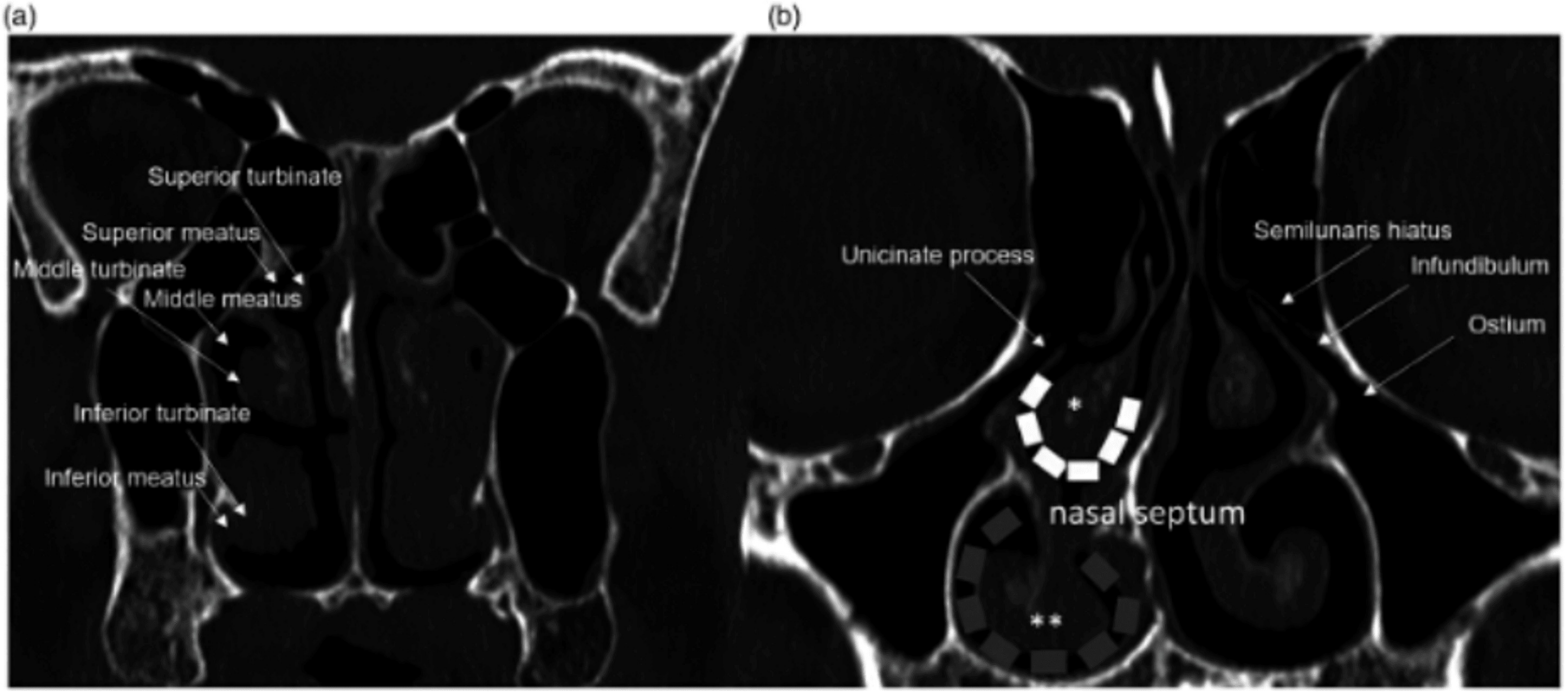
Coronal CT views of the nasal cavity anatomy and turbinate-related obstruction (a) Right nasal cavity showing normal anatomical structures: inferior, middle, and superior turbinates and their respective meatuses. The left nasal cavity is obstructed due to nasal polyposis. (b) Key anatomical landmarks for sinus ventilation and drainage, including the uncinate process, semilunar hiatus, infundibulum, and ostium. A white dashed line indicates the middle meatus; a grey dashed line outlines the inferior meatus; a single asterisk denotes the middle turbinate; and a double asterisk indicates the inferior turbinate. Figure reproduced from Cellina M, Gibelli D, Cappella A, Martinenghi C, Belloni E, Oliva G. *Nasal cavities and the nasal septum: Anatomical variants and assessment of features with computed tomography.* The Neuroradiology Journal (Volume 33, Issue number 4). pp. 340-7. Copyright © 2020, Sage Publications. Reproduced with permission of Sage Publications [11].
